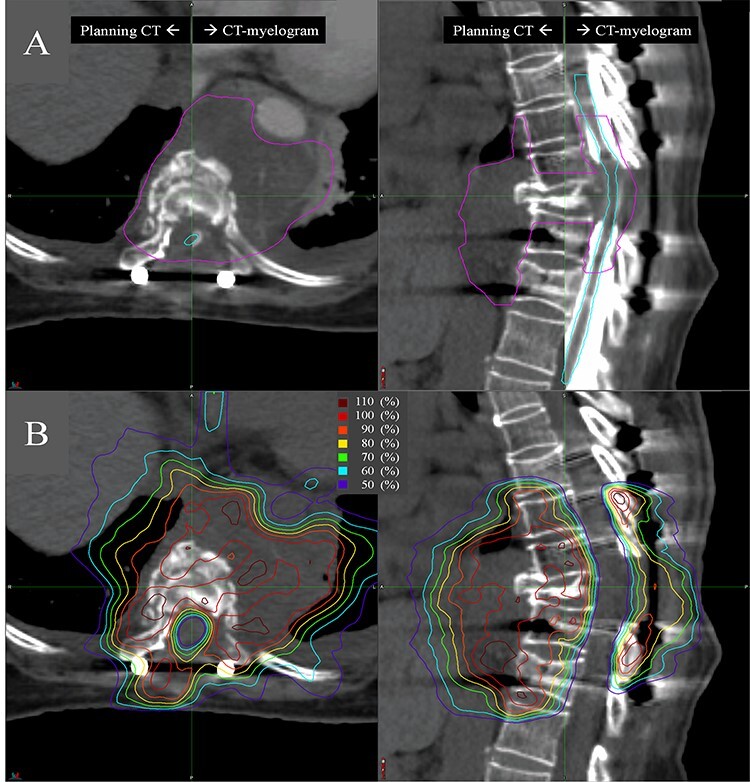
An example of treatment planning for postoperative spine SBRT. A 59-year-old woman with metastatic endometrial cancer was affected in the ninth thoracic vertebrae and surrounding epidural/prevertebral space. A: Fused CT images of planning CT (left) and CT-myelogram (right) in the axial and sagittal plane with delineated clinical target volume (pink) and spinal cord (light blue). B: Axial and sagittal plane of planning CT with dose distribution.
